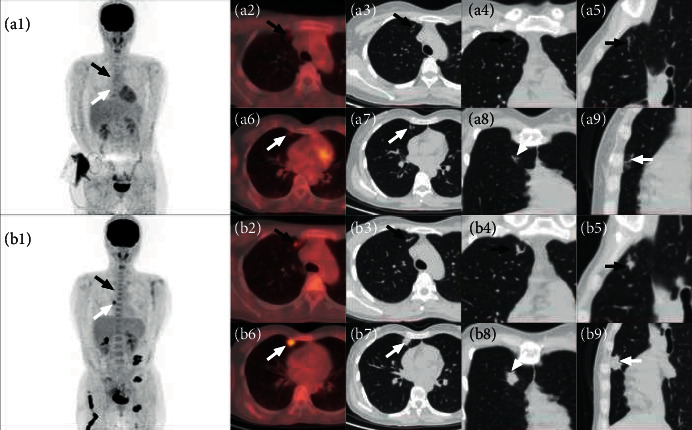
A 30-year-old woman with cervical cancer underwent surgery. ^18^F-FDG PET/CT (a1-2 and a6) scan showed an increased uptake of FDG in the right upper lobe of the lung subpleura. The SUVmax were 1.5 (white arrow) and 1.2 (black arrow), respectively. HRCT (a3-5 and a7-9) revealed corresponding branch-like enlargement of pulmonary blood vessels. After 6 months of follow-up of ^18^F-FDG PET/CT (b1-2 and b6) and HRCT (b3-5 and b7-9), two lesions were found to be enlarged in volume and increased in metabolism. The SUVmax were 6.5 and 3.0, respectively. The lesions below (shown by white arrow) were enlarged as masses.
